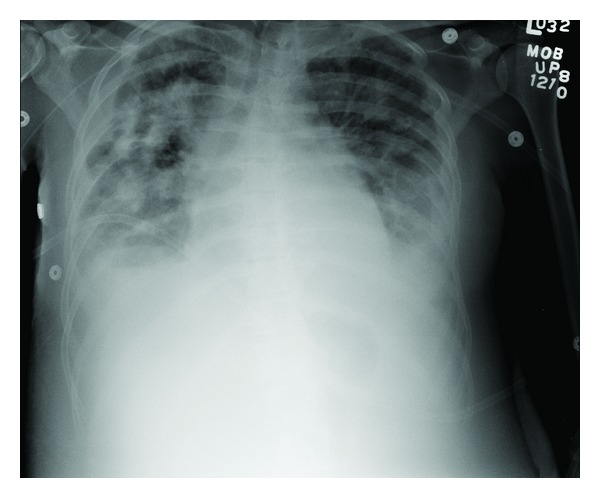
Chest X-ray showing progression of diffuse pulmonary infiltrates.
